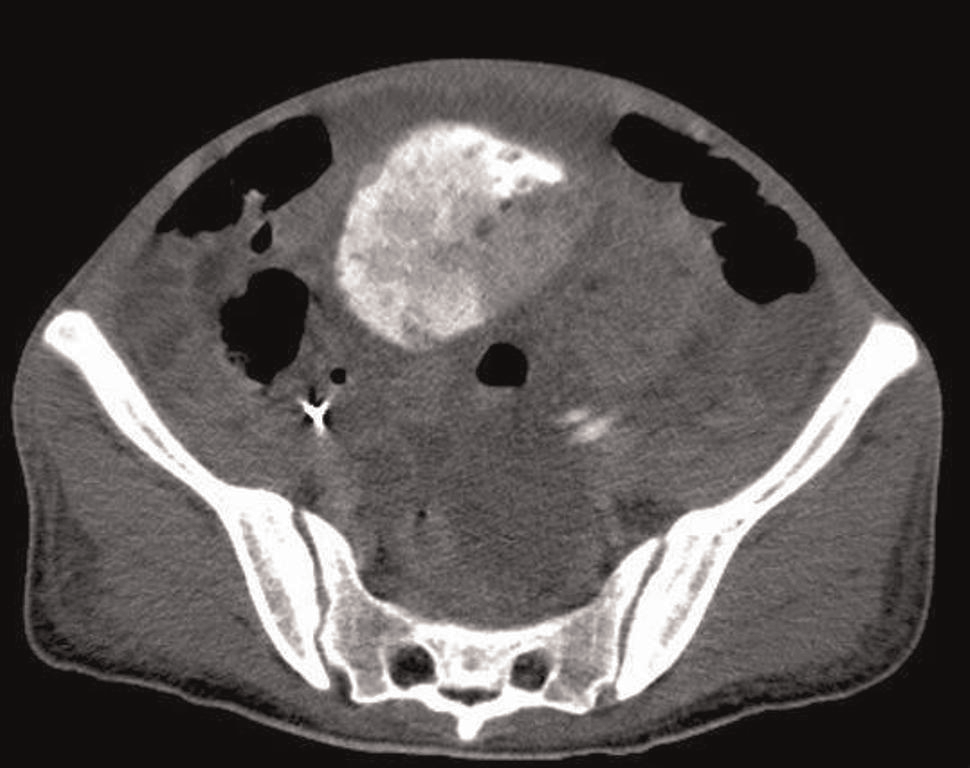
CT during selective arteriography. Most of the normal renal parenchyma can be visualized, allowing precise identification of the feeding blood vessels of the normal kidney, although there are filling defects in a part of the normal kidney.
